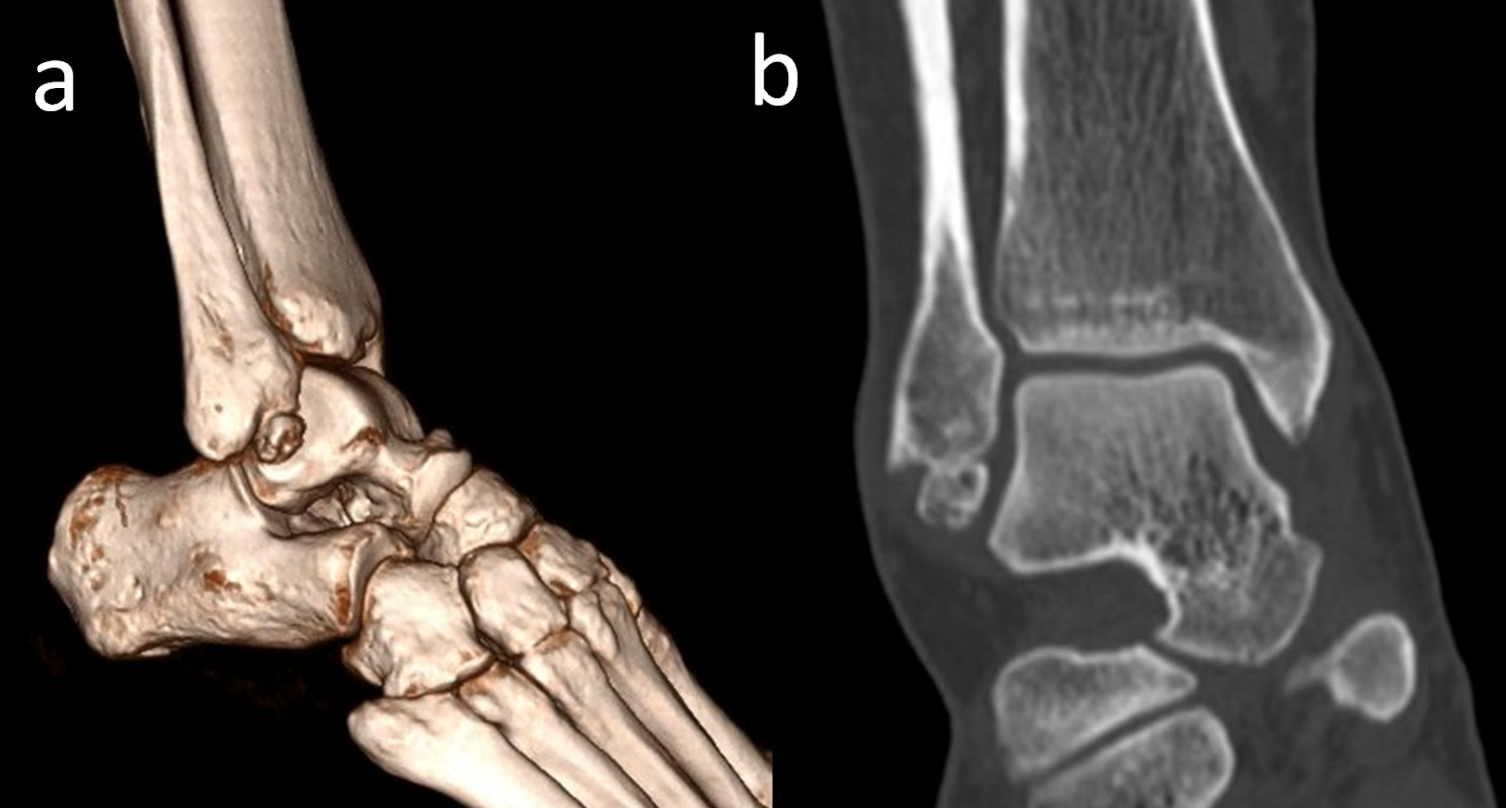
A 42-year-old male patient with a united anterior talofibular ligament avulsion fracture (a) Union is seen on coronal computed tomography (CT); (b) 2D CT appearance.
